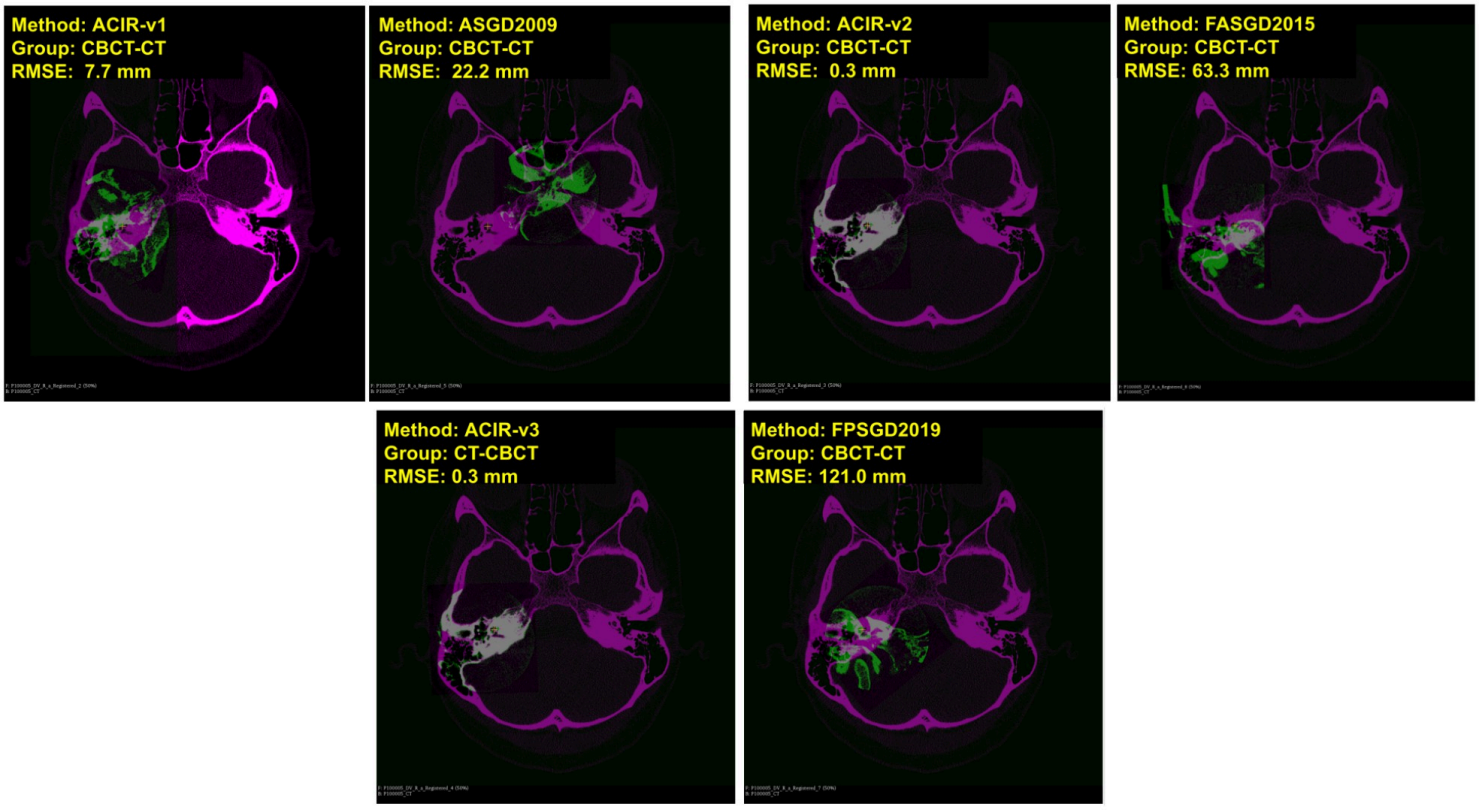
Sample results of registration CBCT to MDCT (axial views). The figure shows image types, methods, and RMSE information. Magenta represents the fixed image and green represents the moving image.
